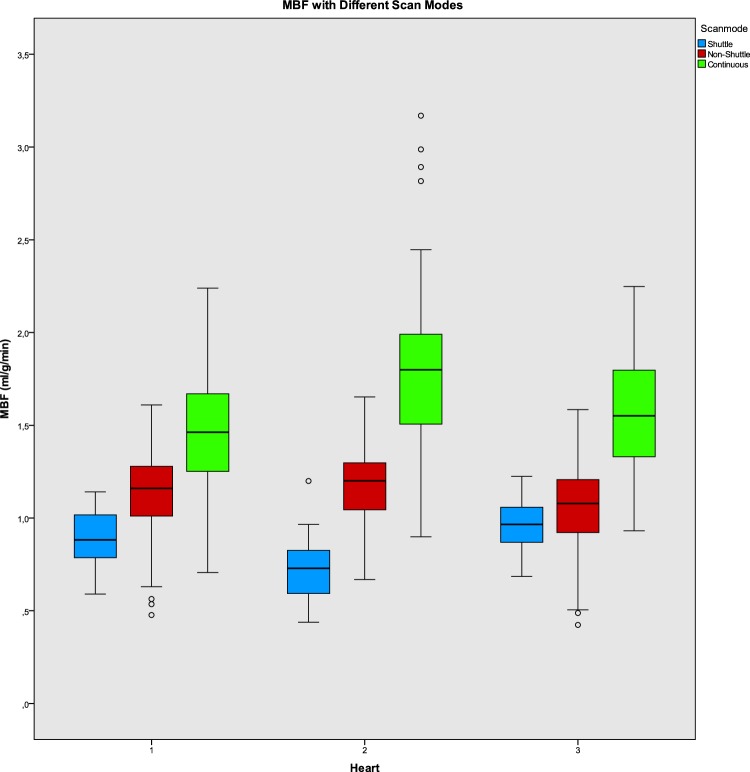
The median myocardial blood flow (MBF) in mL/min/g and interquartile range (IQR) for non-ischemic segments per heart for shuttle, non-shuttle and continuous CT perfusion scan mode
